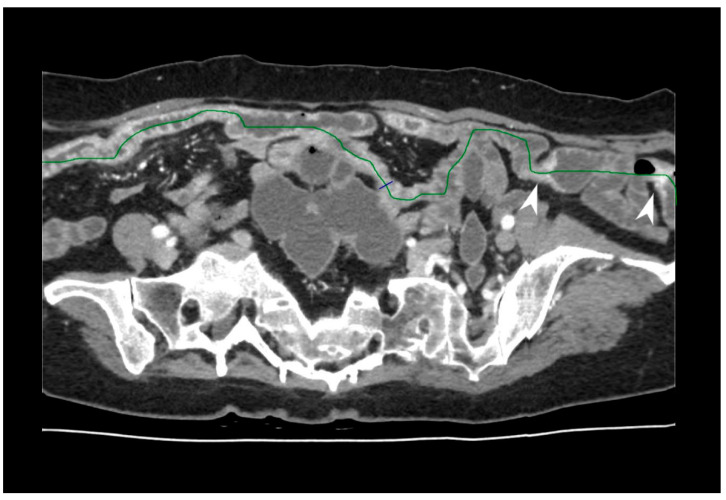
Calculation of the length of affected intestine using postprocessing software; a tubular view of the small bowel loops from CT-E is shown. There is evidence of two areas of pathological wall thickening on the right of the picture (arrowheads). The green line indicates the center of the bowel lumen. Original figures by LMM and LL.
